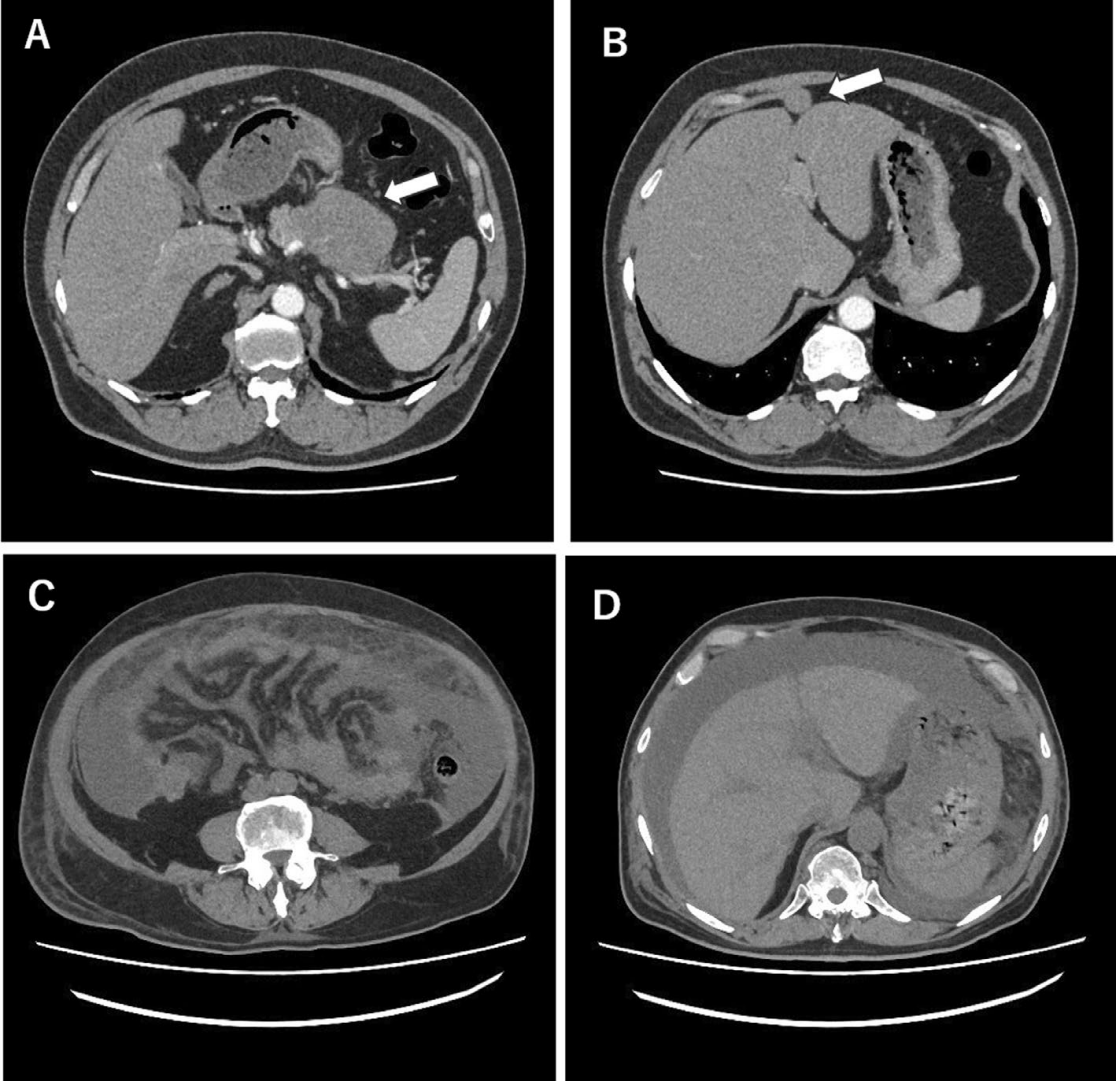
Abdominal computerized tomography imagings at the first presentation and plasmablastic transformation (Case 2). An abdominal CT imaging of obtained at the first presentation of Case 2 showed a noninvasive mass in the head of the pancreas (Panel A, indicated by an arrow) and multiple masses in the peritoneum (Panel B, indicated by an arrow). An abdominal CT imaging of obtained after local irradiation therapy for the head of the pancreas of Case 2 showed pan‐peritonitis (Panel C) and massive ascites (Panel D).
